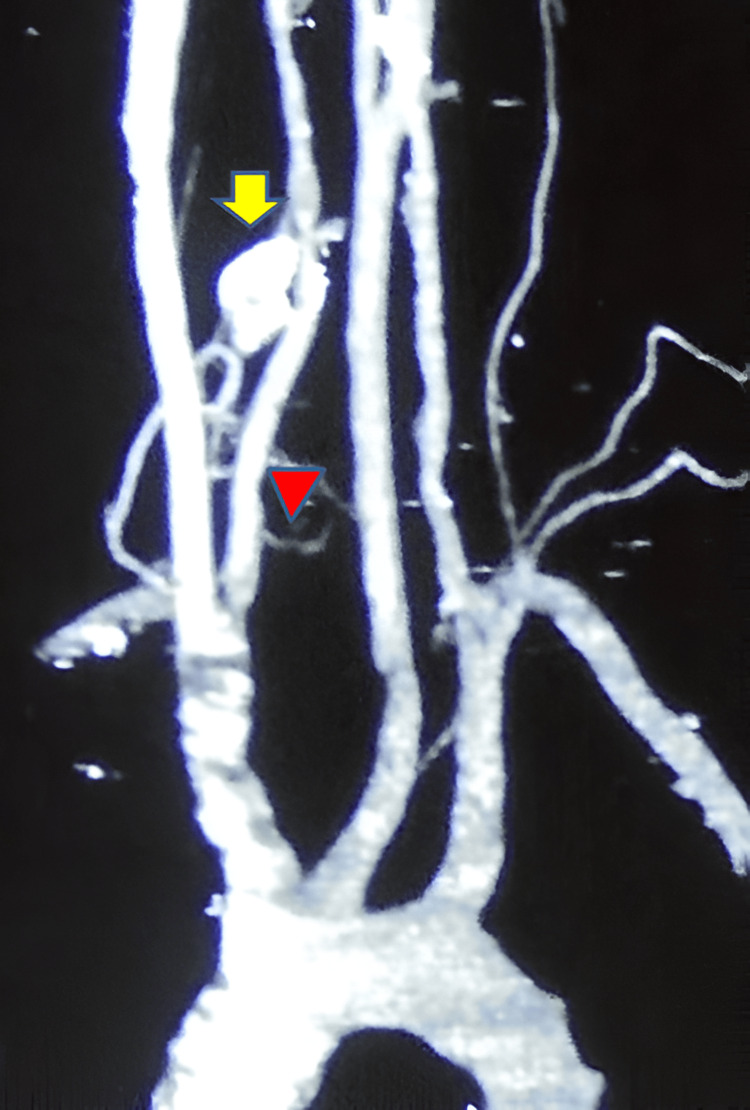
Three-dimensional reconstructed CT angiography with MIP image showing the tumor (arrow). The arrowhead indicates the right vertebral artery, which is encroached upon and displaced by the tumor, highlighting the critical vascular relationship important for surgical planning CT: computed tomography; MIP: maximum intensity projection
